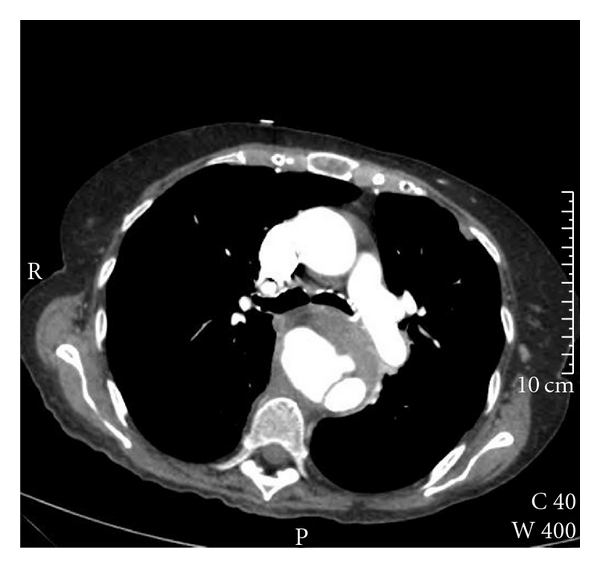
CT scan of the thoracic aorta demonstrating a mycotic aneurysm in the descending thoracic aorta.
